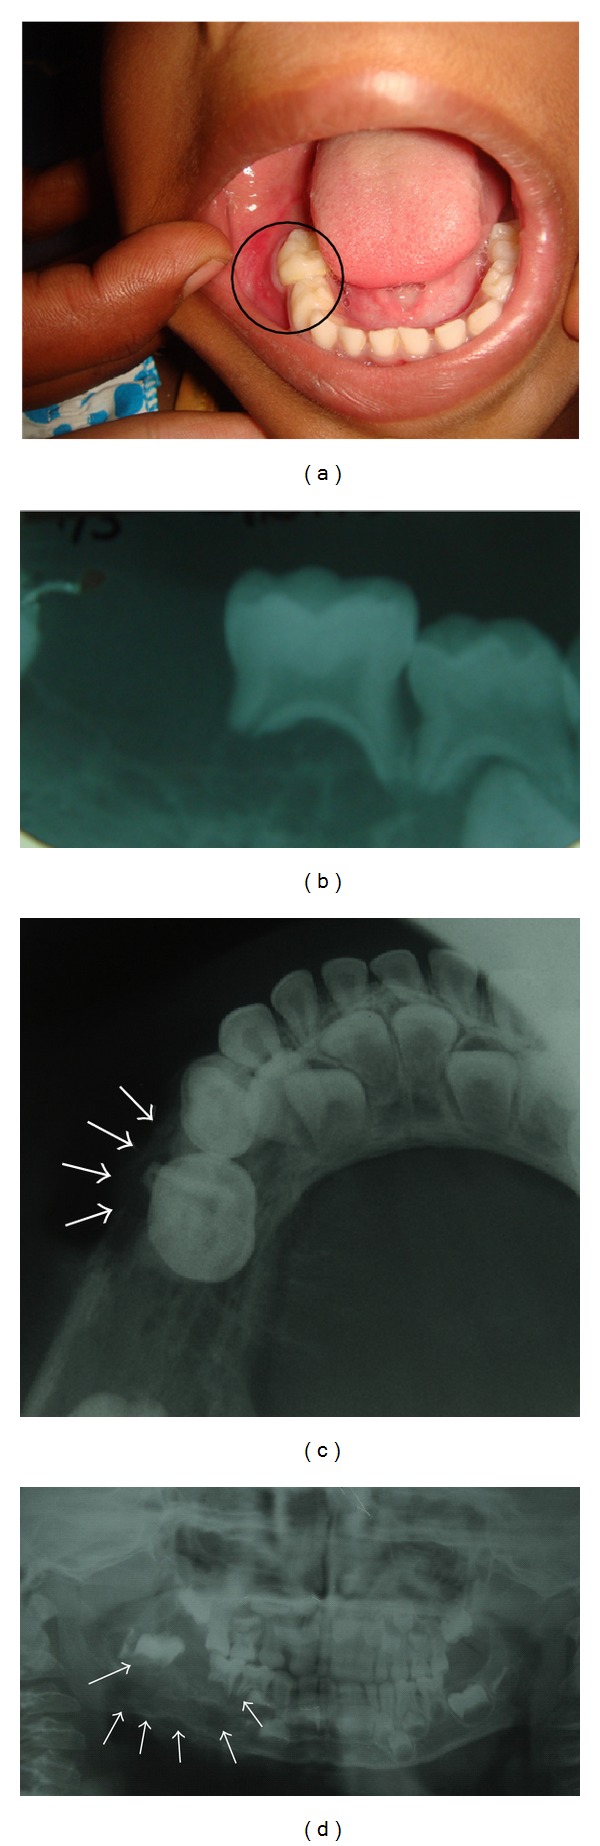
(a) Erythema of the vestibular mucosa in relation to 84 and 85. (b) Intraoral periapical radiograph showing resorption of roots of 84, 85, periapical radiolucency, and displaced tooth buds. (c) Mandibular occlusal radiograph showing erosion of the buccal cortex in relation to 85. (d) Panoramic radiograph showing lytic lesion over the right mandible. Note the irregular lower border of the mandible along with the displaced tooth bud of 46 (arrows).
